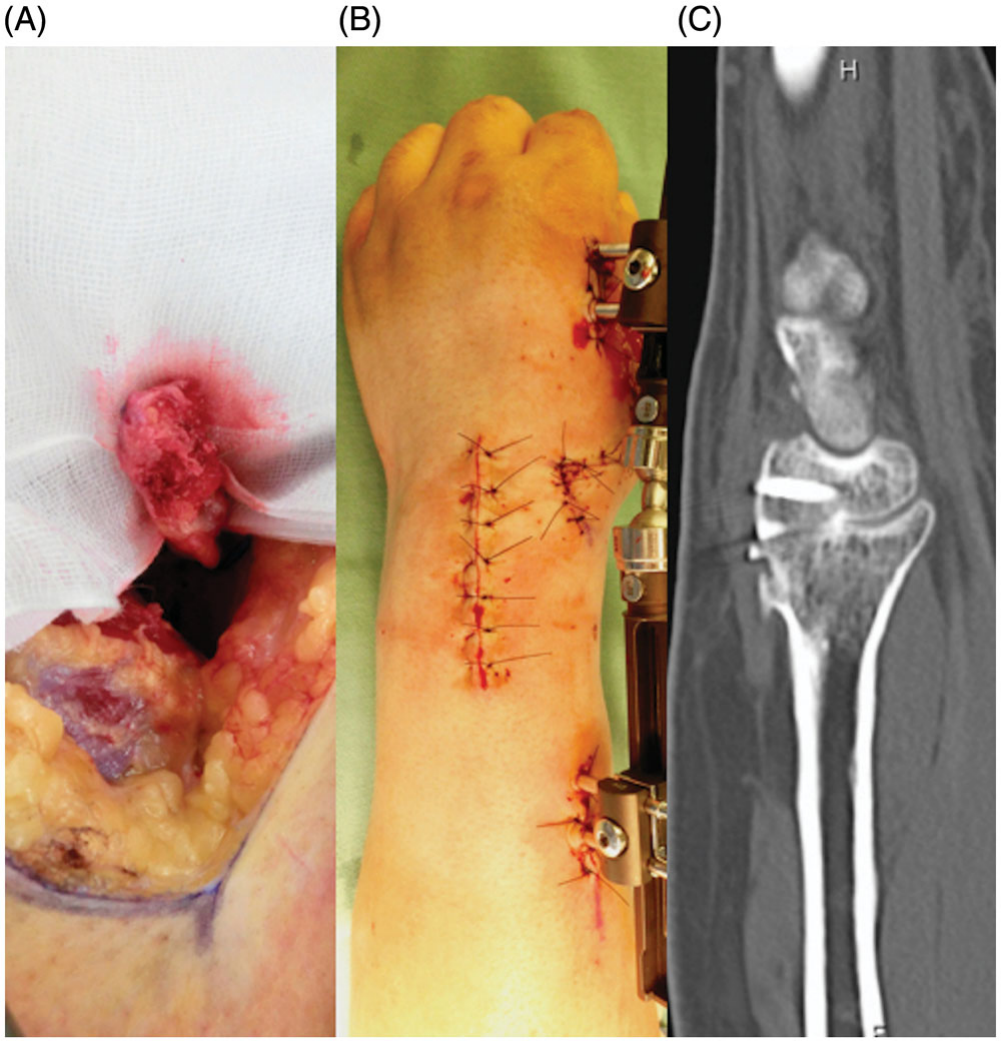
(A) Intraoperative photograph of the vascularized bone graft at the time of harvesting. (B) Immediately postoperative photograph of the wrist with the external fixation device. (C) At 3 months after the last surgery, a radiograph confirmed bony union.
